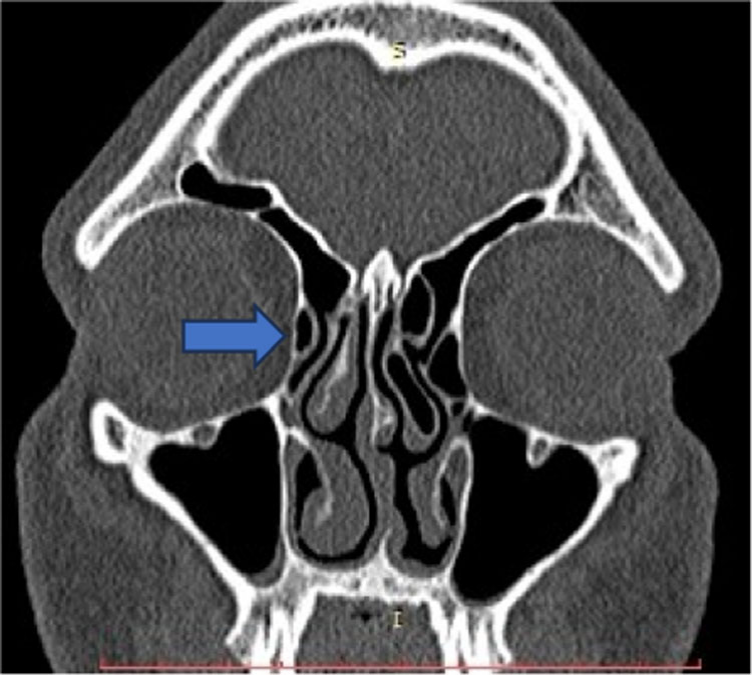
CT coronal image shows agger nasi cell in ethmoid sinus.
